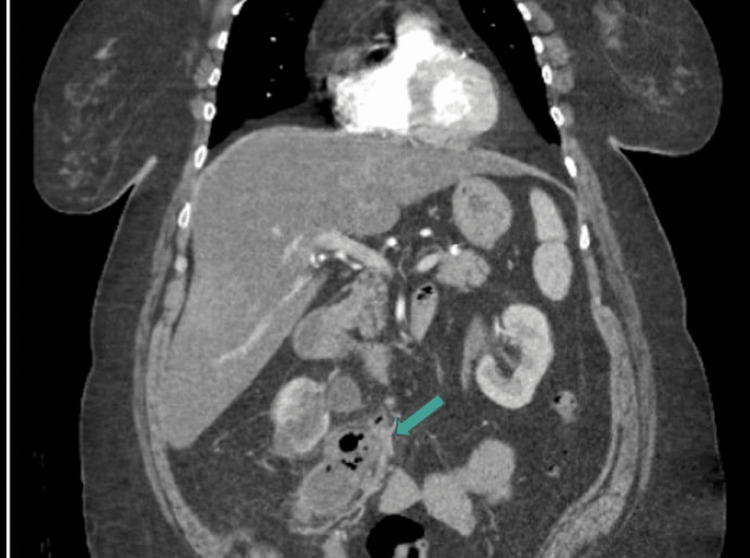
CT coronal view of gas and fluid-filled peri-ureteral structure, consistent with abscess CT: computed tomography
